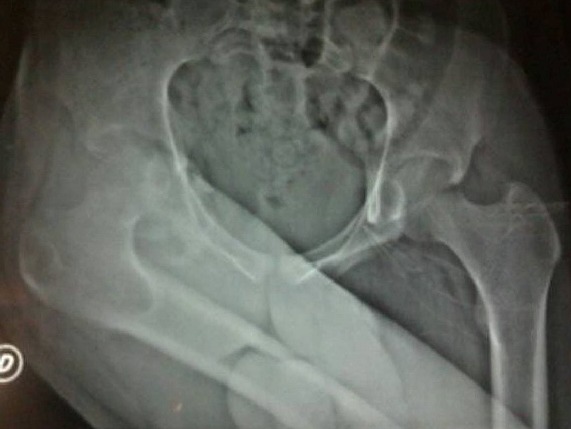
Radiographie standard du bassin montant une luxation négligée de la hanche
